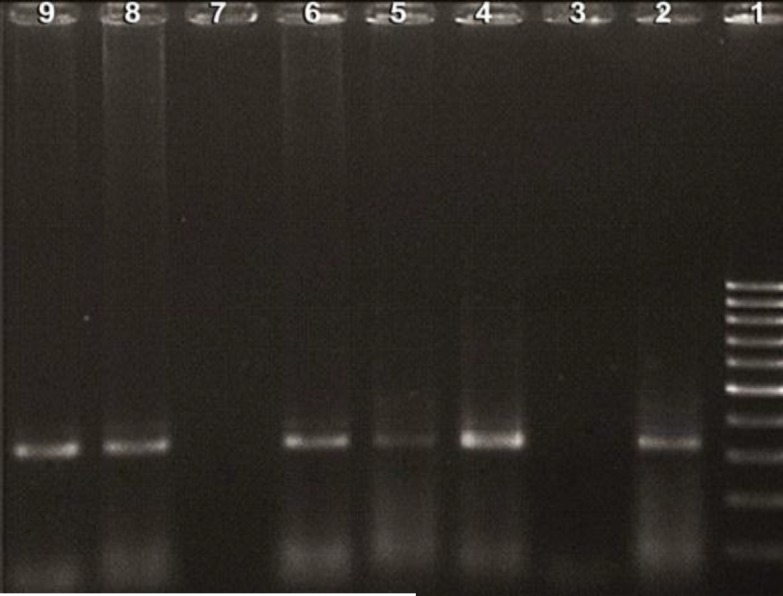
Agarose gel electrophoresis analysis of CT PCR products.
